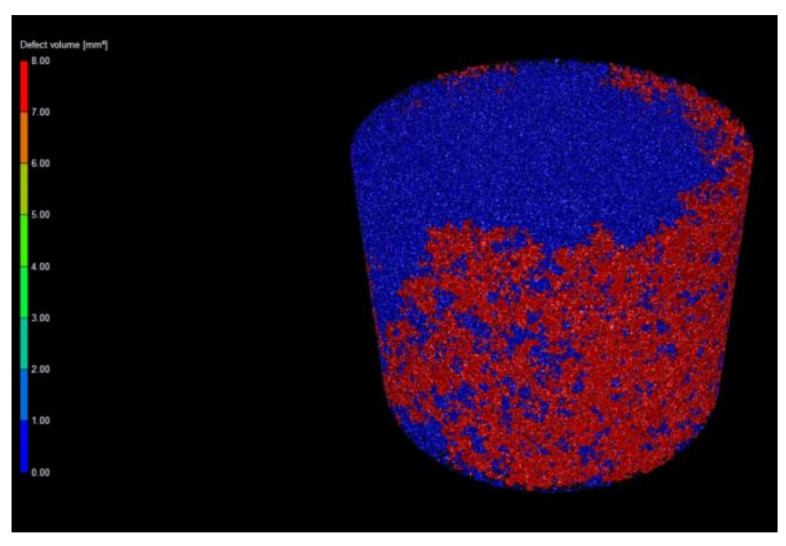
The Cu–graphite sample with 90 vol. % graphite investigated using X-ray tomography: a surface cluster of copper is in red; volumetric copper cluster is in blue (sample diameter is 8 mm).
